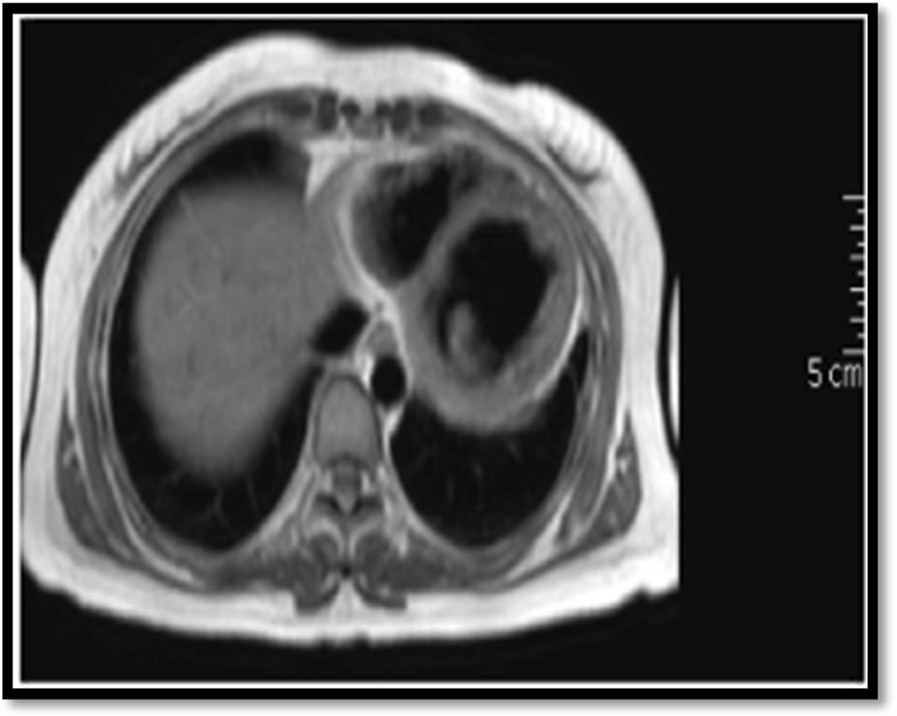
Cardiac magnetic resonance imaging showing subepicardial late gadolinium enhancement of the basal inferior, anteroseptal, and inferoseptal segments. The findings indicate dilated cardiomyopathy with previous myocarditis (not active anymore, indicated by lack of myocardial edema)
